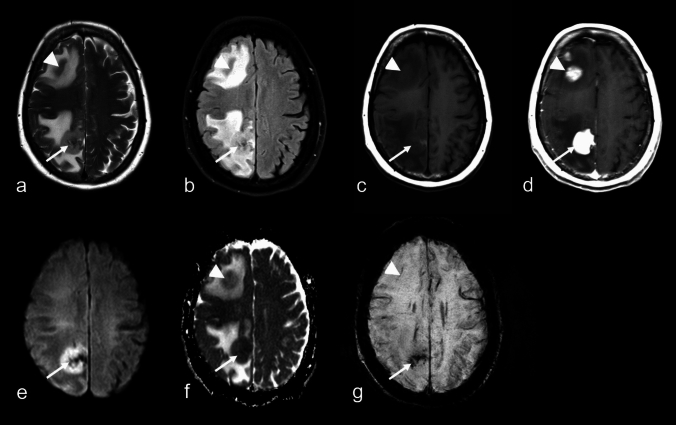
56-year-old woman with primary large B-cell lymphoma of immune-privileged sites (IP-LBCL), negative for Epstein-Barr virus. MRI (a: T2-weighted imaging (WI); b: fluid-attenuated inversion recovery; c, d: T1WI; e, f: diffusion-WI, apparent diffusion coefficient (ADC) map) reveal two lesions, one in the right frontal lobe (arrowheads) and one in the right parietal lobe (arrows), with perifocal edema and nearly homogeneous contrast enhancement (d). Susceptibility-WI shows confluent signal loss in the parietal lesion (g; arrow), while the frontal lesion exhibits no definite tumor-associated signal loss (g; arrowhead: linear signal loss corresponds to a vein). Note the different diffusion alterations between the lesions: parietal ADC mean ± SD: 0.53 ± 0.07 × 10⁻^3^ mm^2^/s (f; arrow); frontal ADC mean ± SD: 0.86 ± 0.14 × 10⁻^3^ mm^2^/s (f; arrowhead)
